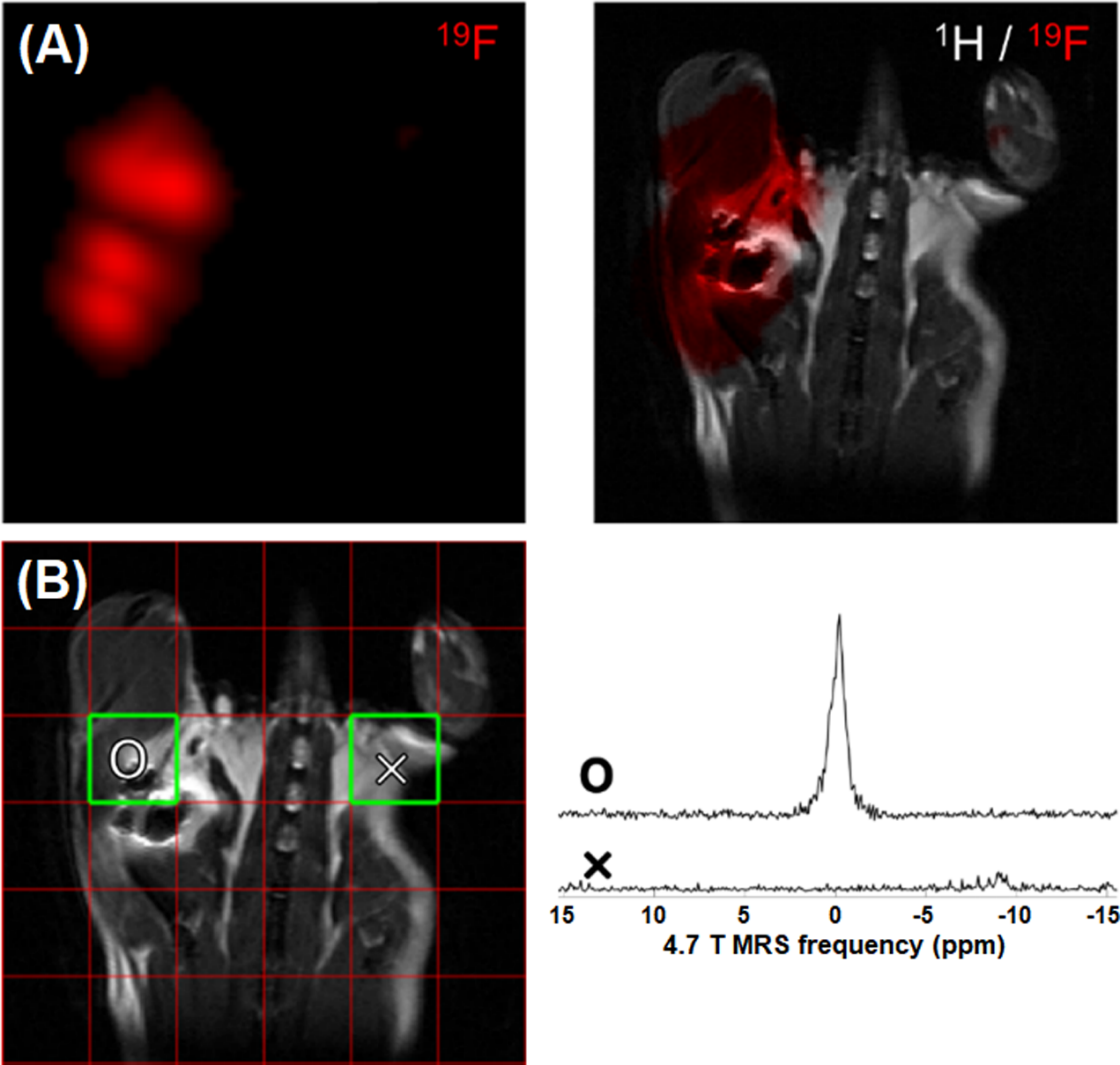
In vivo*^19^F MRS and MRI of F5H2 nanotracer
